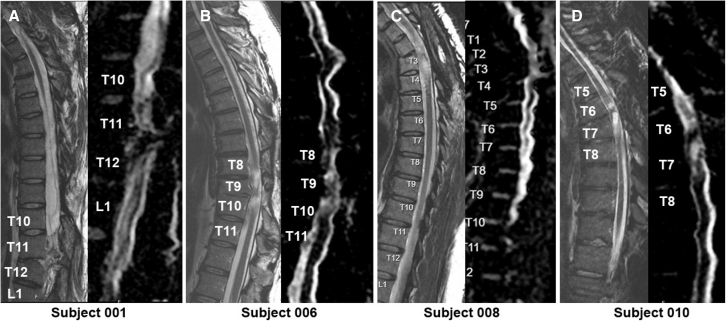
MRI cervical-thoracic T2 sagittal and AvDC sagittal sequences (A) Subject 001 at 60 months (T2) and 18 months (AvDC) post-transplant (myelomalacia is seen at the area of SCI with no extension of myelomalacia or syringomyelia post-transplant), (B) subject 006 at 60 months (T2) and 30 months (AvDC) post-transplant, (C) subject 008 at 24 months (T2, AvDC) post-transplant, and (D) subject 010 at 24 months (T2, AvDC) post-transplant. In all four patients, T2 and AvDC imaging revealed a stable appearance of the spinal cord both at the injury site and rostral/caudal to the injury site.
